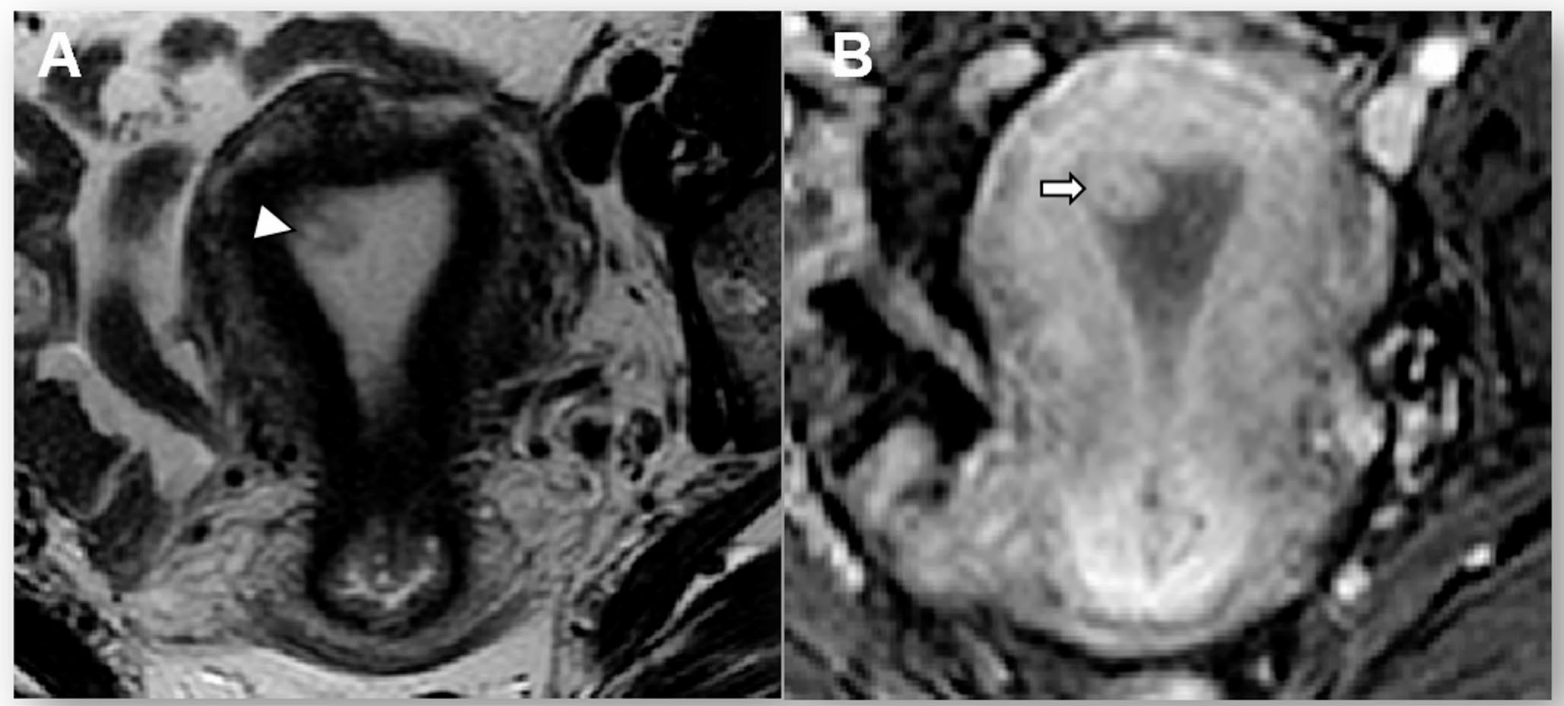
Stage IA1 grade 1 endometrioid carcinoma, no LVSI, in a 48-year-old woman. A Axial oblique T2-weighted fast spin-echo (FSE) image (TR/TE: 4500/100 ms, slice thickness 3 mm) and B contrast-enhanced 3D T1-weighted fat-suppressed LAVA sequence (TR/TE: 4.2/2.1 ms; slice thickness: 2 mm; post-gadolinium, delayed phase at 4 min) show an endometrial polyp without myometrial invasion (arrow). On the T2-WI, hyperintense cystic spaces are seen within the polyp (arrowhead)
